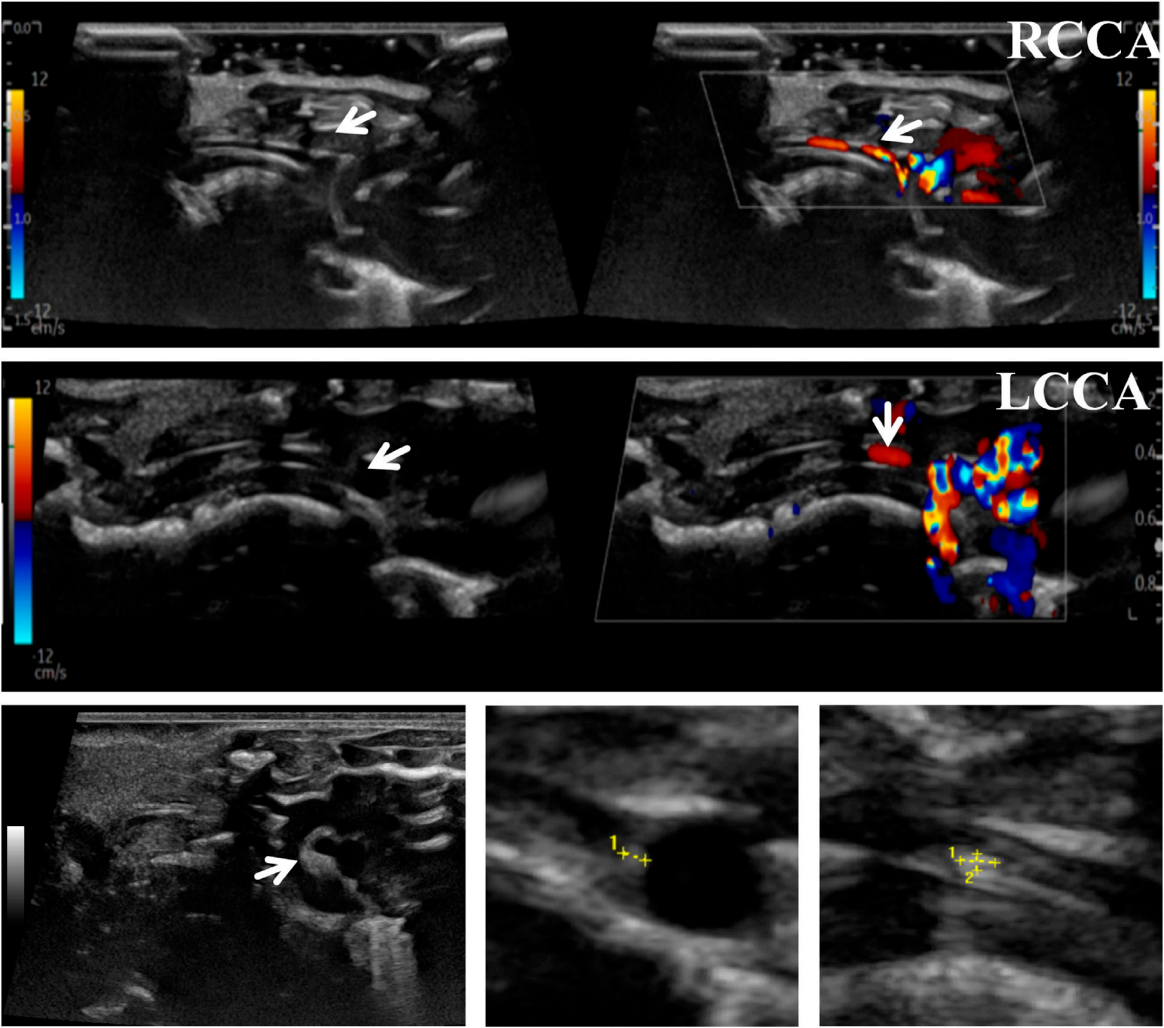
Imaging features of arterial vessels and plaques detected by ultrasound.
